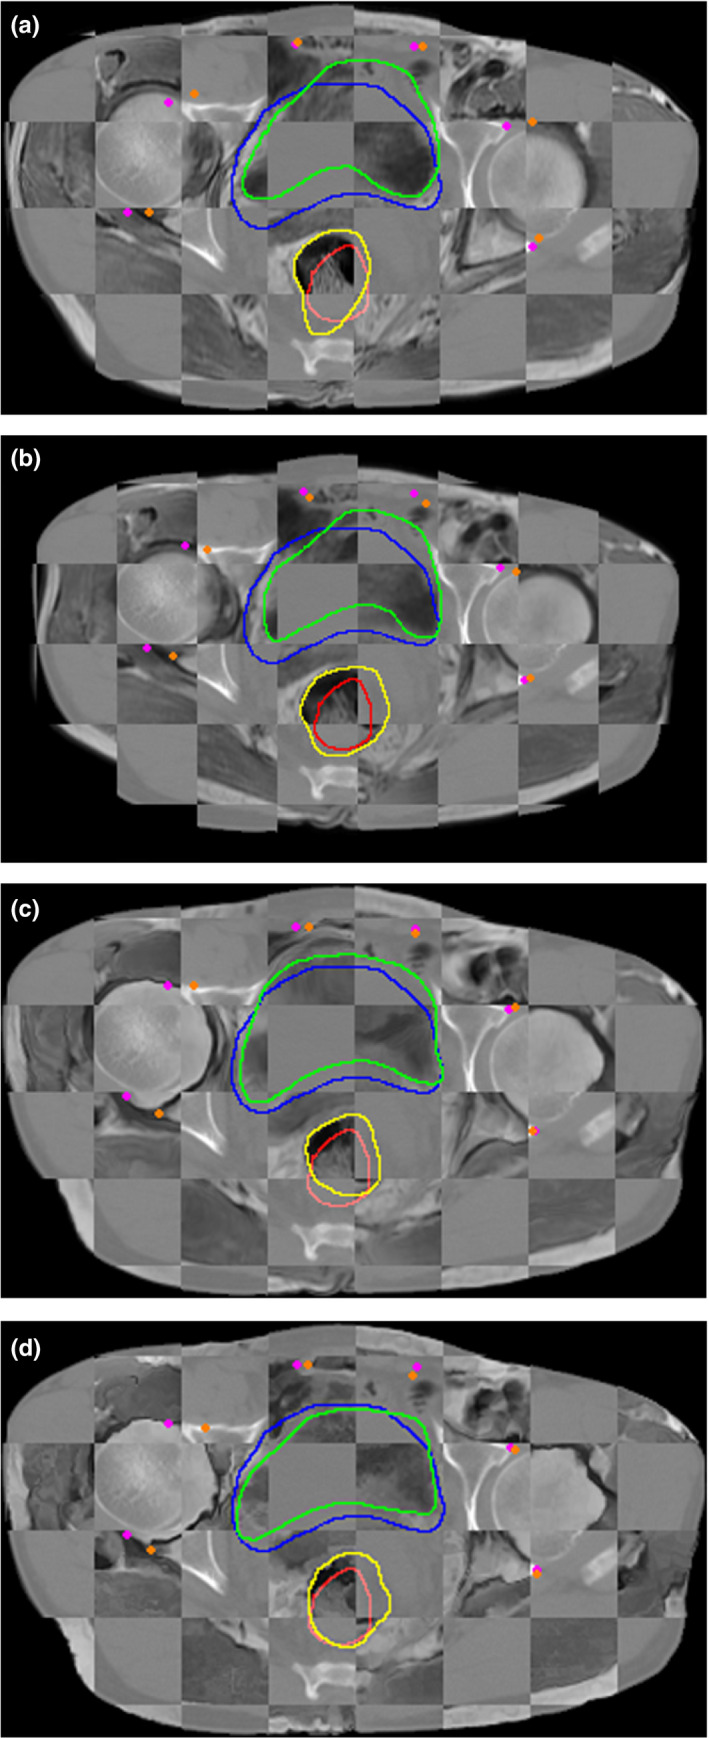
Checkboard fusion images of computed tomography (CT) and magnetic resonance (MR) after registration with different methods. (a) the fusion image using Elastix; (b) the fusion image using MIM; (c) the fusion image using fully convolutional network (FCN) with Cycle‐Consistent; (d) the fusion image using FCN without Cycle‐Consistent. The red, blue, yellow, and green contours represent the rectum of fixed image, the bladder of fixed image, the rectum of deformed moving image, and the bladder of deformed moving image. The purple and orange points represent the corresponding points of CT and deformed MR.
